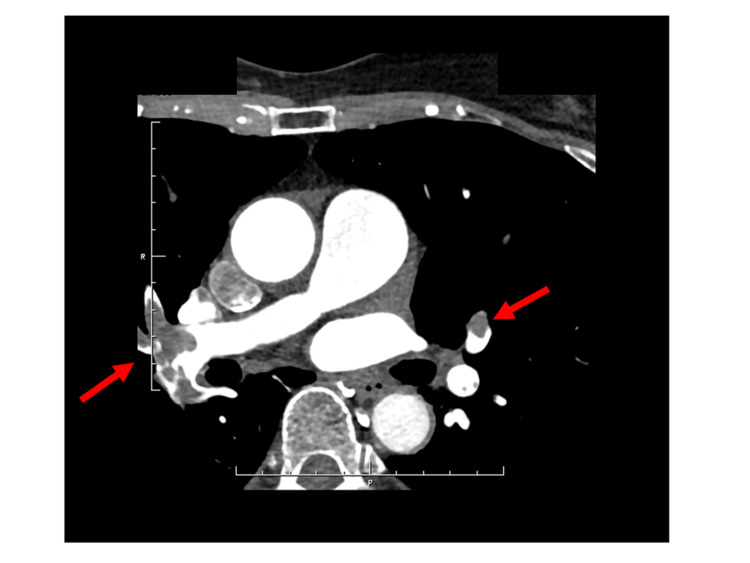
Axial computed tomography angiography of the pulmonary arteries with pulmonary thrombi on both sides Arrows indicate pulmonary thrombi on both sides of the pulmonary arteries.
